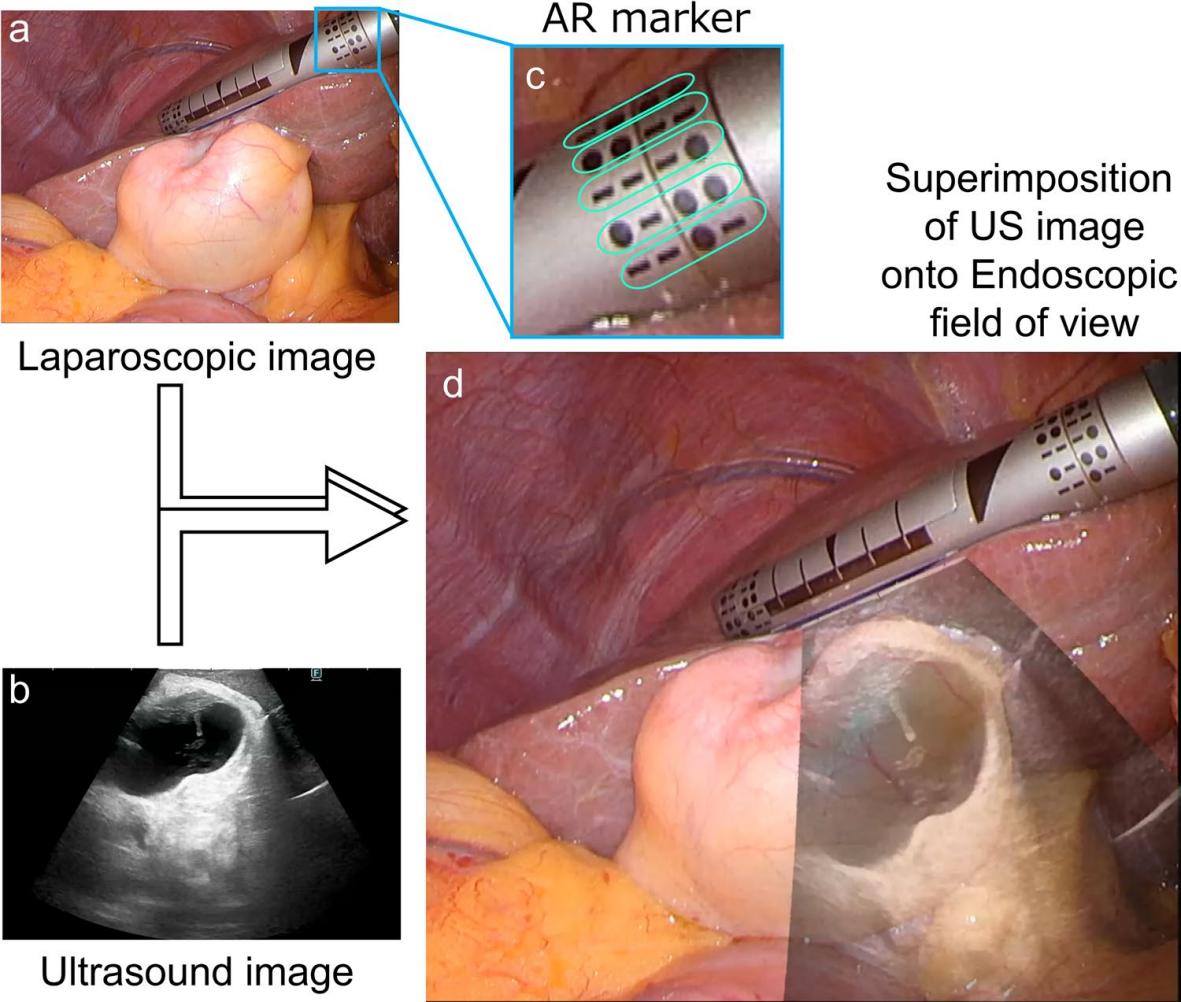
Overview of the Flag AR. a Laparoscopic view showing the L43LAP ultrasound probe positioned on liver surface to examine gallbladder tumor during minimally invasive surgery. b Corresponding ultrasound image investigating inside of gallbladder and gall bladder tumor. c Magnified view of the AR marker system on the probe tip, showing 13 distinct pattern rows arranged circumferentially around the probe shaft. Each pattern row consists of four symbols with unique sequence configurations. d Real-time ultrasound image superimposition onto the laparoscopic field of view using Flag AR technology, with the ultrasound image overlaid at 1:1 scale
